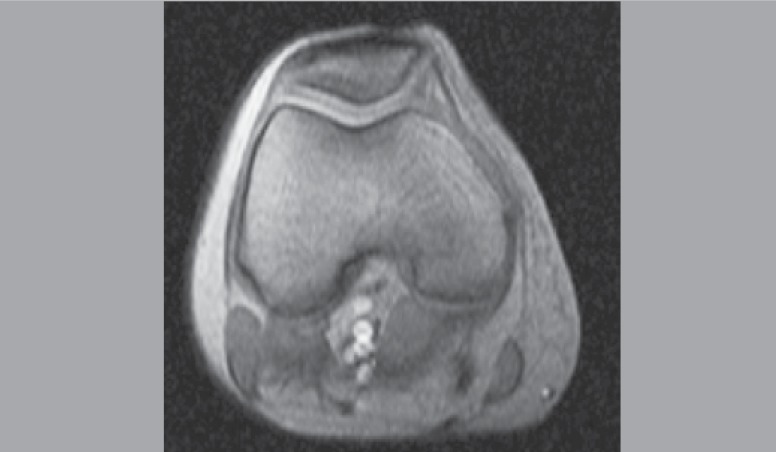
MRI Image of patella in axial plan with higher patellar latero- -medial diameter.
